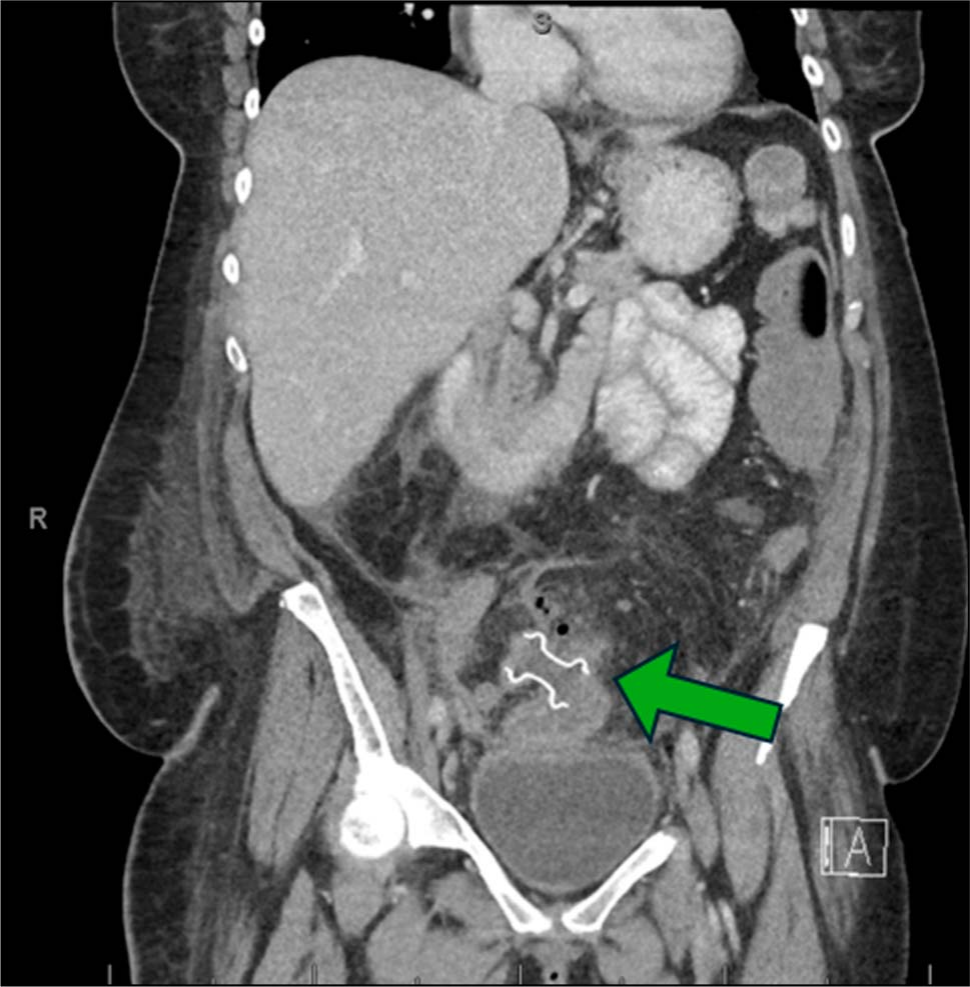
Coronal contrast-enhanced computed tomography showing interval decompression of the orphaned bowel loop after stent placement, with reduced luminal distention and no evidence of perforation or new collection (green arrow).
